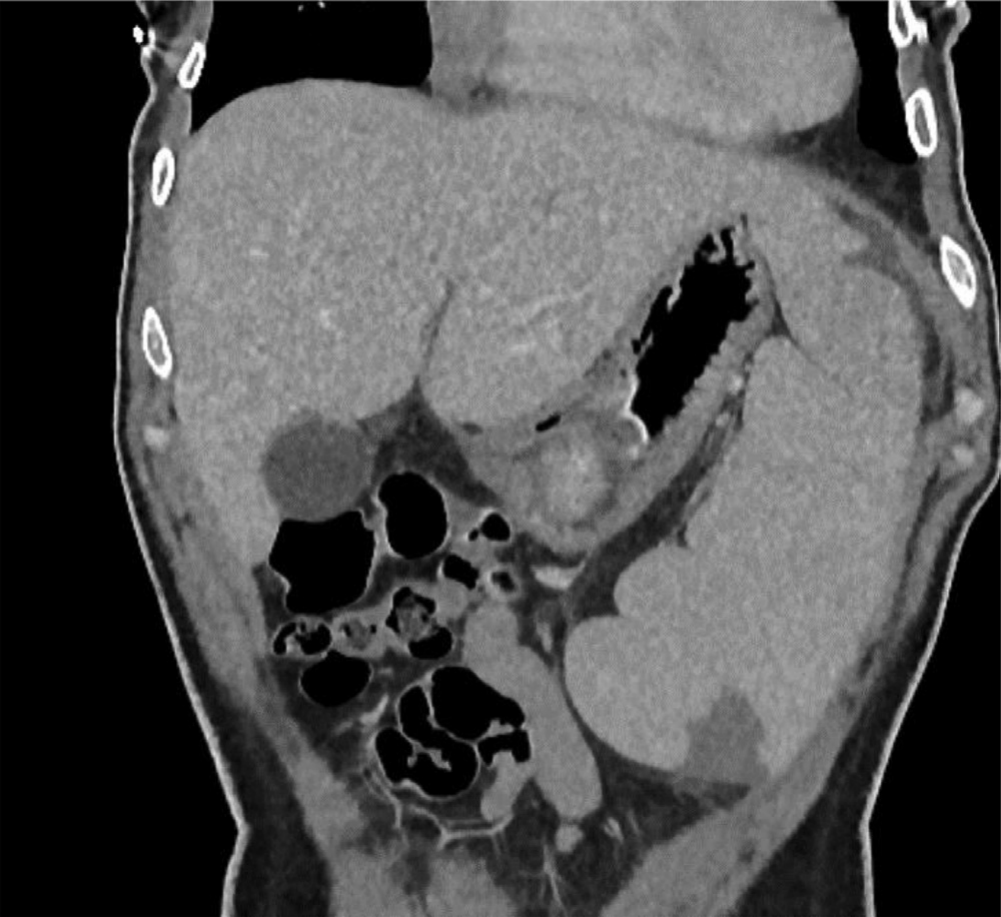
Abdomen CT scan revealed wedge-shaped splenic infarction.
